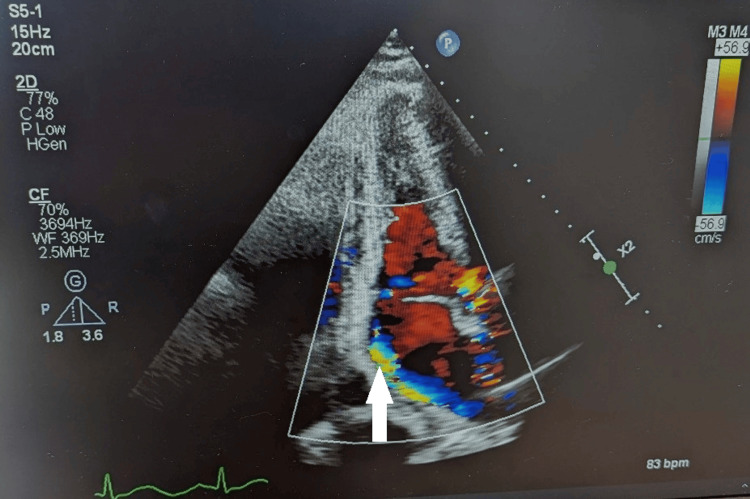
TTE, apical two-chamber view, with colour flow Doppler (Nyquist limit 56.9 cm/s) Demonstrating a large eccentric mitral regurgitation (MR) jet (mosaic colour signal- marked as white arrow) filling the majority of the left atrium (LA) during systole, consistent with severe MR. LV: left ventricle; LA: left atrium; MV: mitral valve. Large mobile vegetations on the aortic and mitral valves were identified on other acquired views and confirmed on transoesophageal echocardiography (TTE).
